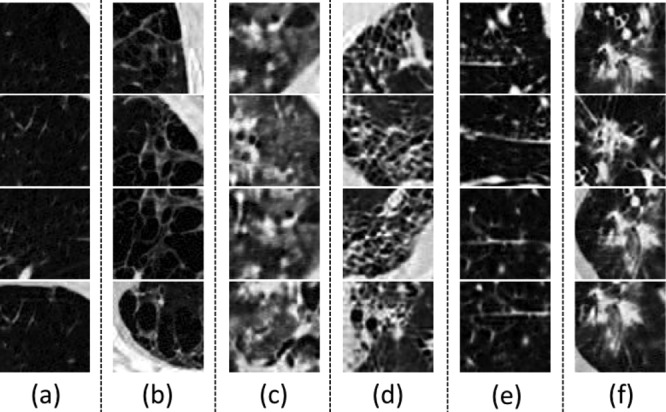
Some examples of }{}$64\times 64~{\rm pixel}$ CT image patches for (a) Nm, (b) Em, (c) Gg, (d) Fb, (e) MN (f) CD.
